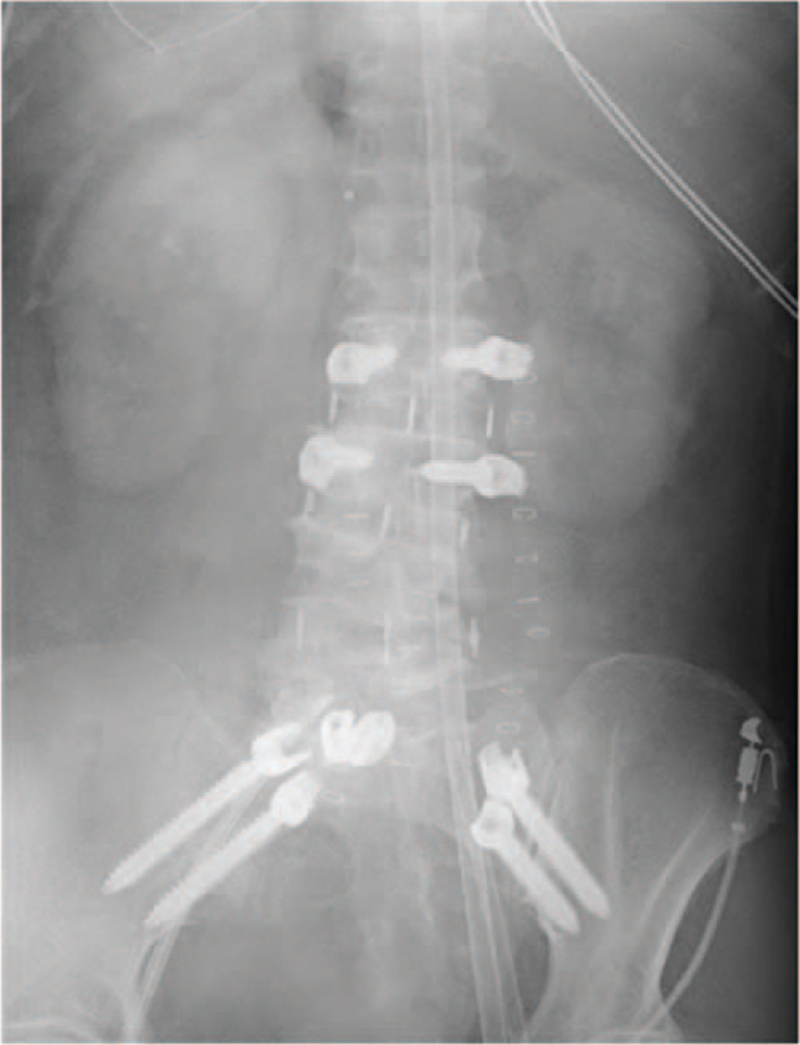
Lumbar X-ray at four days after anterior lumbar interbody fusion. During placement of the L5/S1 interbody fusion cage and iliac screws, followed by the insertion of a pedicle screw with augmented allografted bone, the procedure was interrupted for cardiopulmonary resuscitation due to shock vitals.
